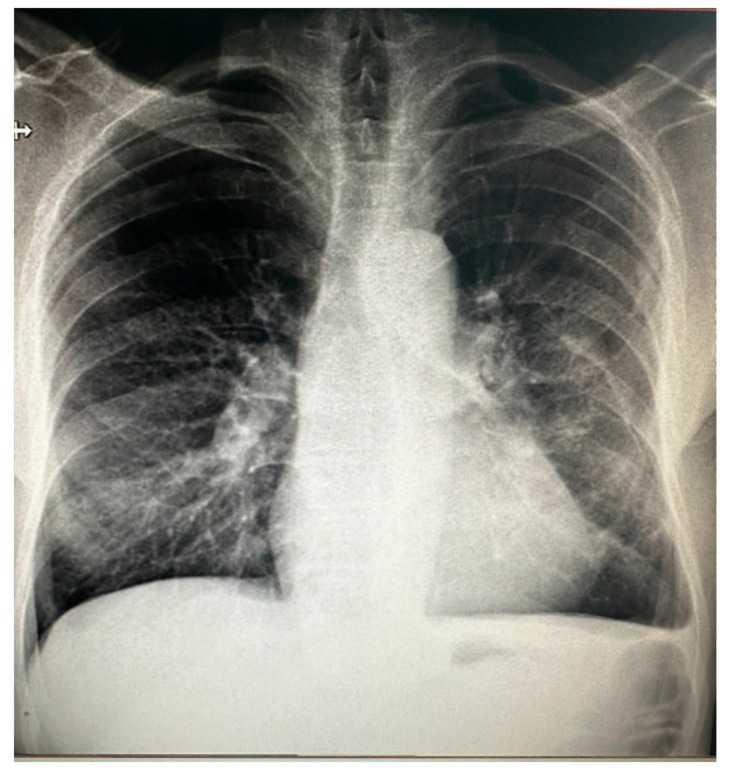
Posterior–anterior chest X-ray.
